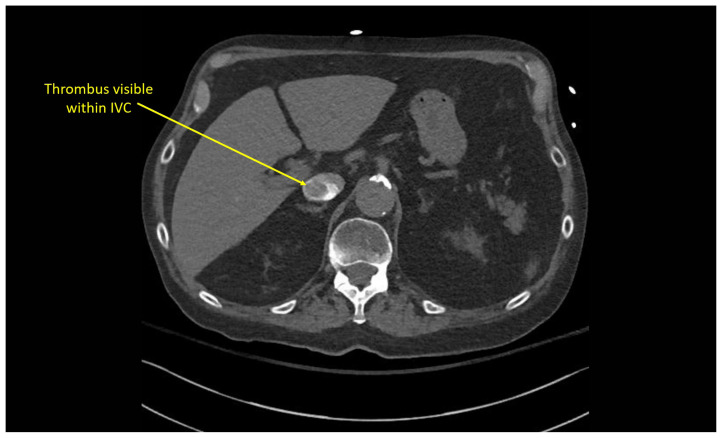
Thrombus extending caudally within the IVC CT Video Link: https://youtu.be/AsTNVTL3sJg
